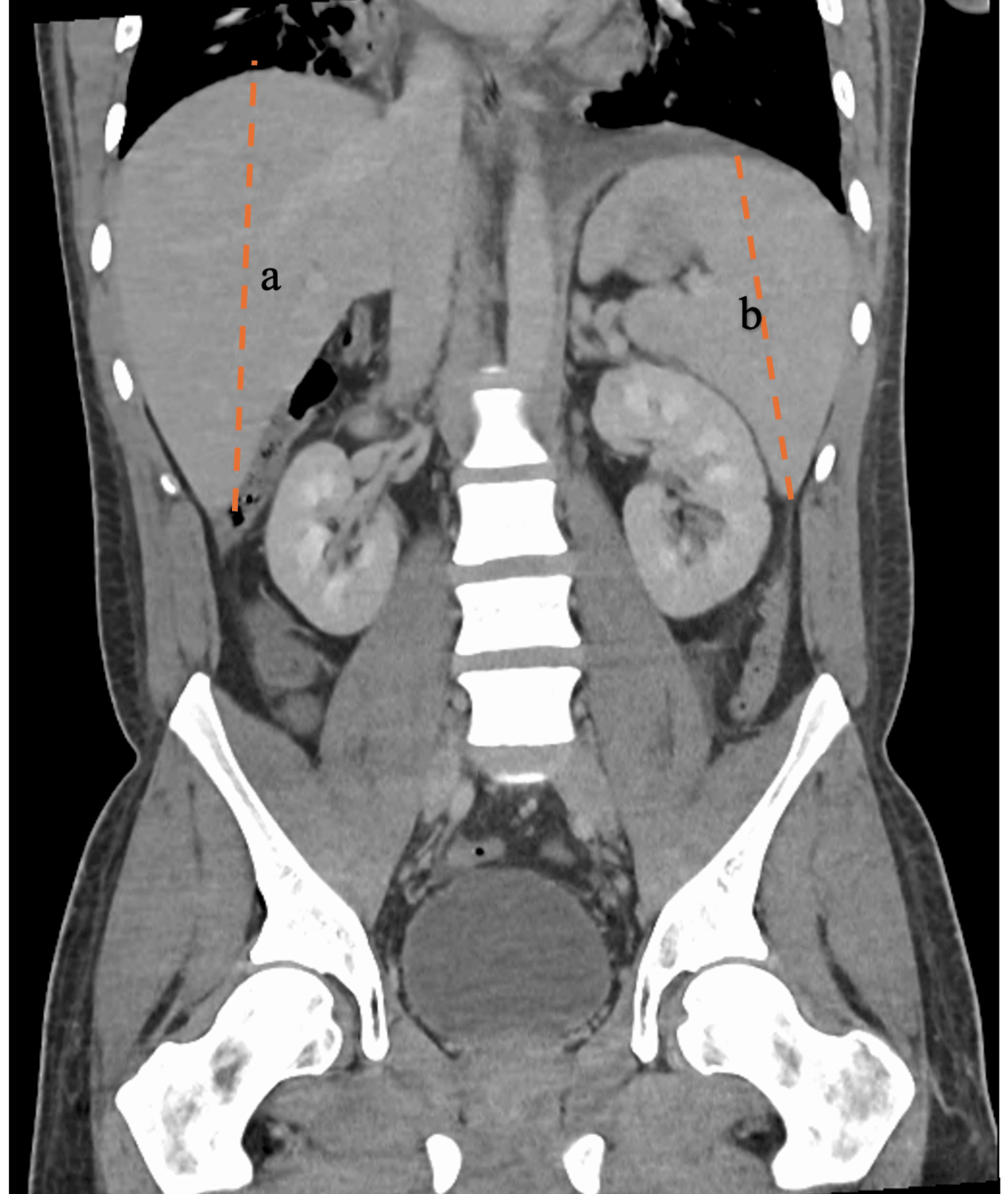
CT scan of abdomen, coronal view, showing hepatomegaly (a, 188 mm), splenomegaly (b, 154 mm)
